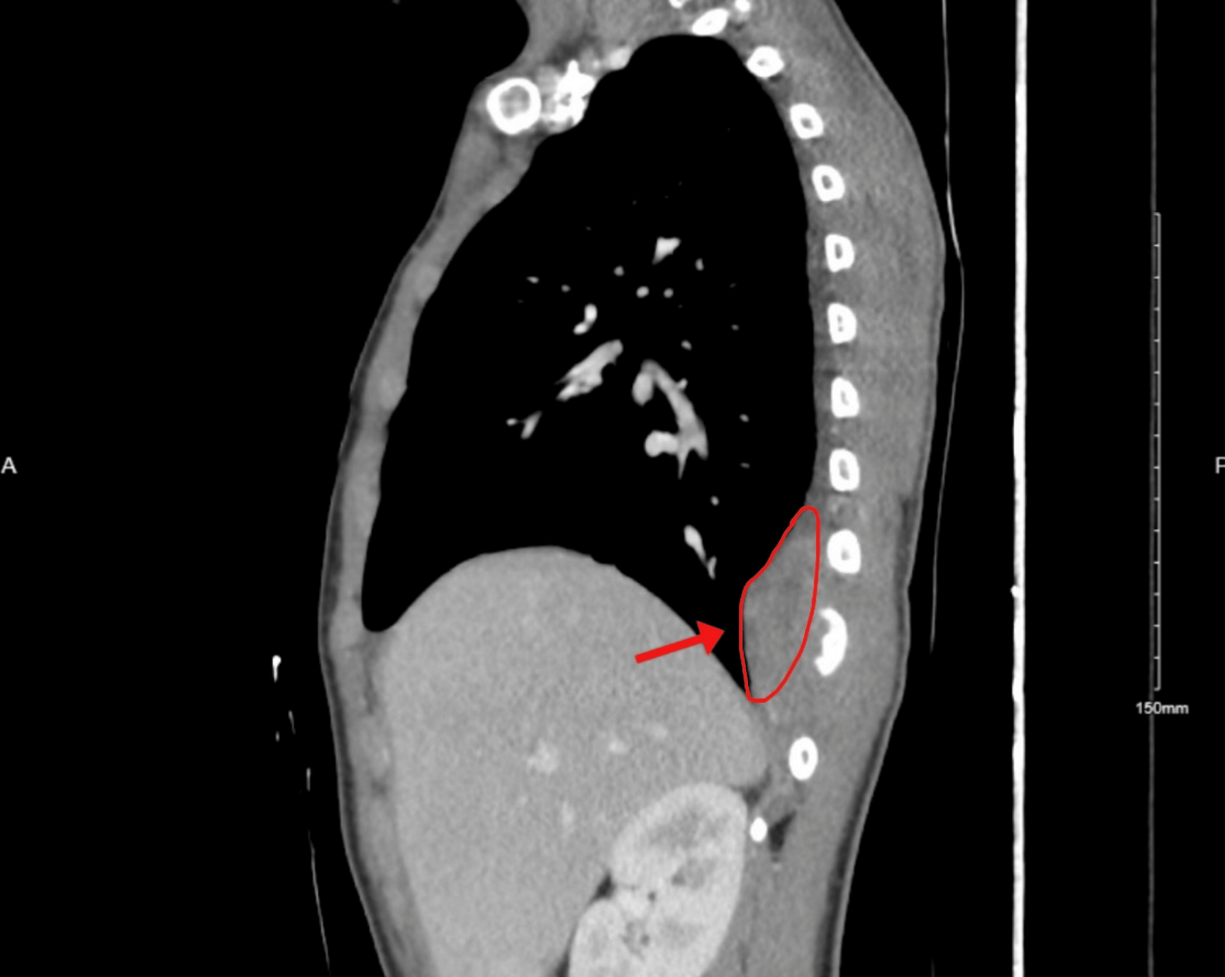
Chest CT demonstrating posterior chest wall lesion
